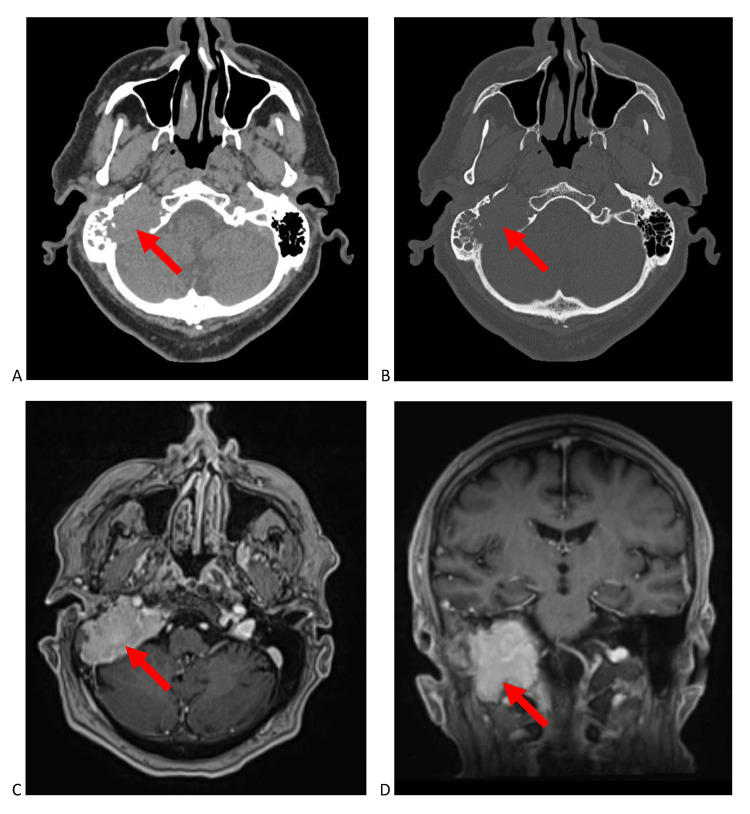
Brain imaging of the tumour in the right jugular foramen at three months in the absence of treatment. (A) soft tissue axial non-contrast computed tomography (CT) and (B) bone-window axial non-contrast CT showing marked interval enlargement of the tumour and further osteolysis. (C) axial and (D) coronal T1-weighted post-contrast magnetic resonance imaging (MRI) showing further extension into the mastoid air spaces and extension to the external auditory canal with obliteration of the vestibular apparatus.
